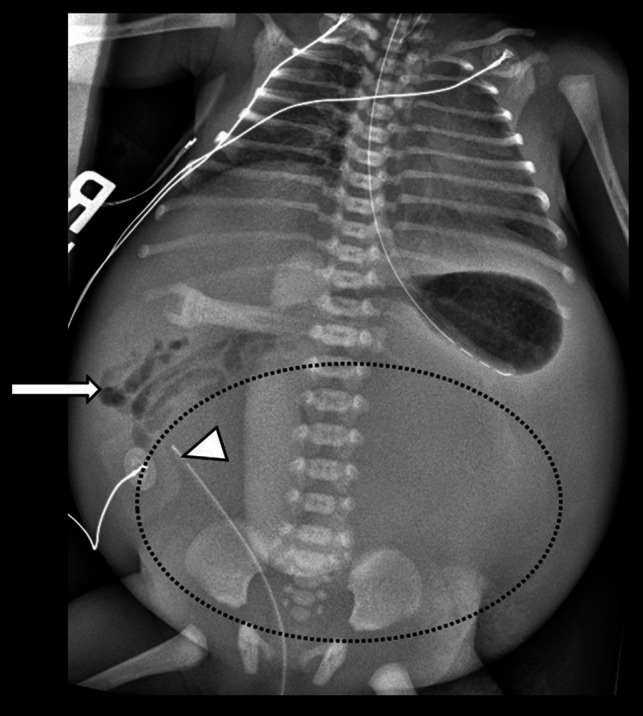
Frontal radiograph of the chest and abdomen on day of life 0 in a newborn girl shows gas-filled loops of bowel confined to the right side of the abdomen (*arrows*), raising concern for malrotation. The tip of the bladder catheter extends above the pelvis (*arrowheads*), and soft tissue density is seen in the pelvis and abdomen (*circle*). These findings indicate massive bladder enlargement
